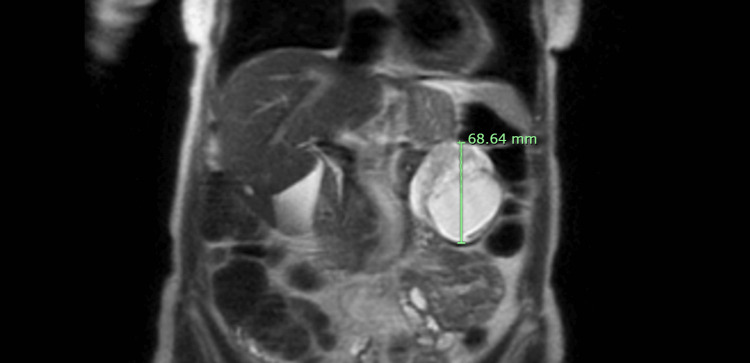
MRI appearance: coronal SS-FSE sequence MRI of the abdomen without contrast (T2-weighted SS-FSE) demonstrating an alternate view of a complex mixed solid and cystic mass with peripheral enhancement and restricted diffusion, possibly arising from the anterior aspect of the pancreatic tail but also apparently continuous with the posterior stomach. SS-FSE, single-shot fast spin-echo; MRI, magnetic resonance imaging
